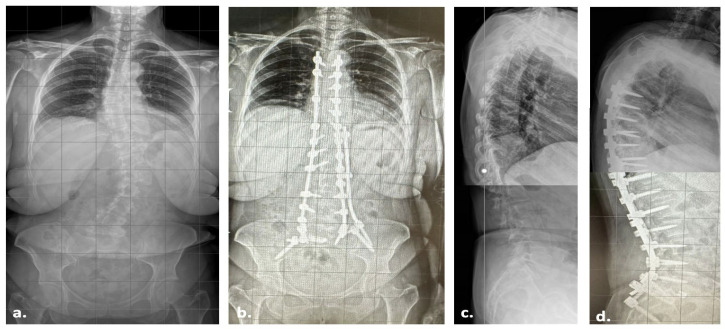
Pre- and post-operative coronal and sagittal standing position X-rays of the 61-year old female patient reported. Preoperative coronal (a), postoperative coronal (b), preoperative sagittal (c), and postoperative sagittal (d).
